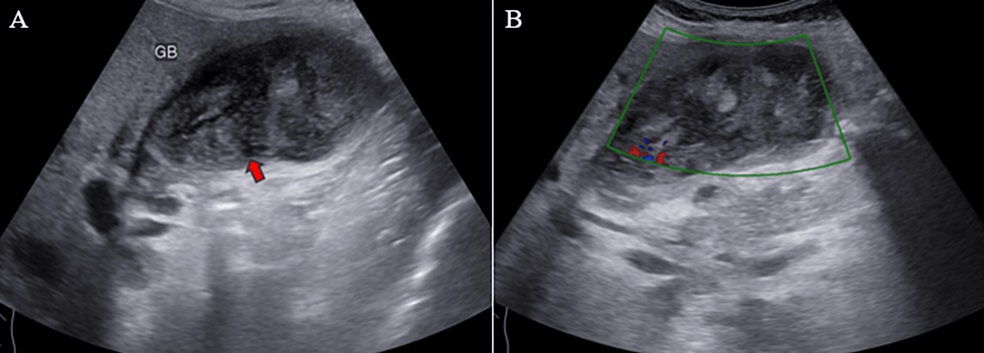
Ultrasonography images demonstrate heterogeneously hypoechoic content (red arrow) in the gallbladder (A) and absent vascularity within the content on color Doppler (B). The peripheral vascularity observed is artifactual. GB: Gallbladder
